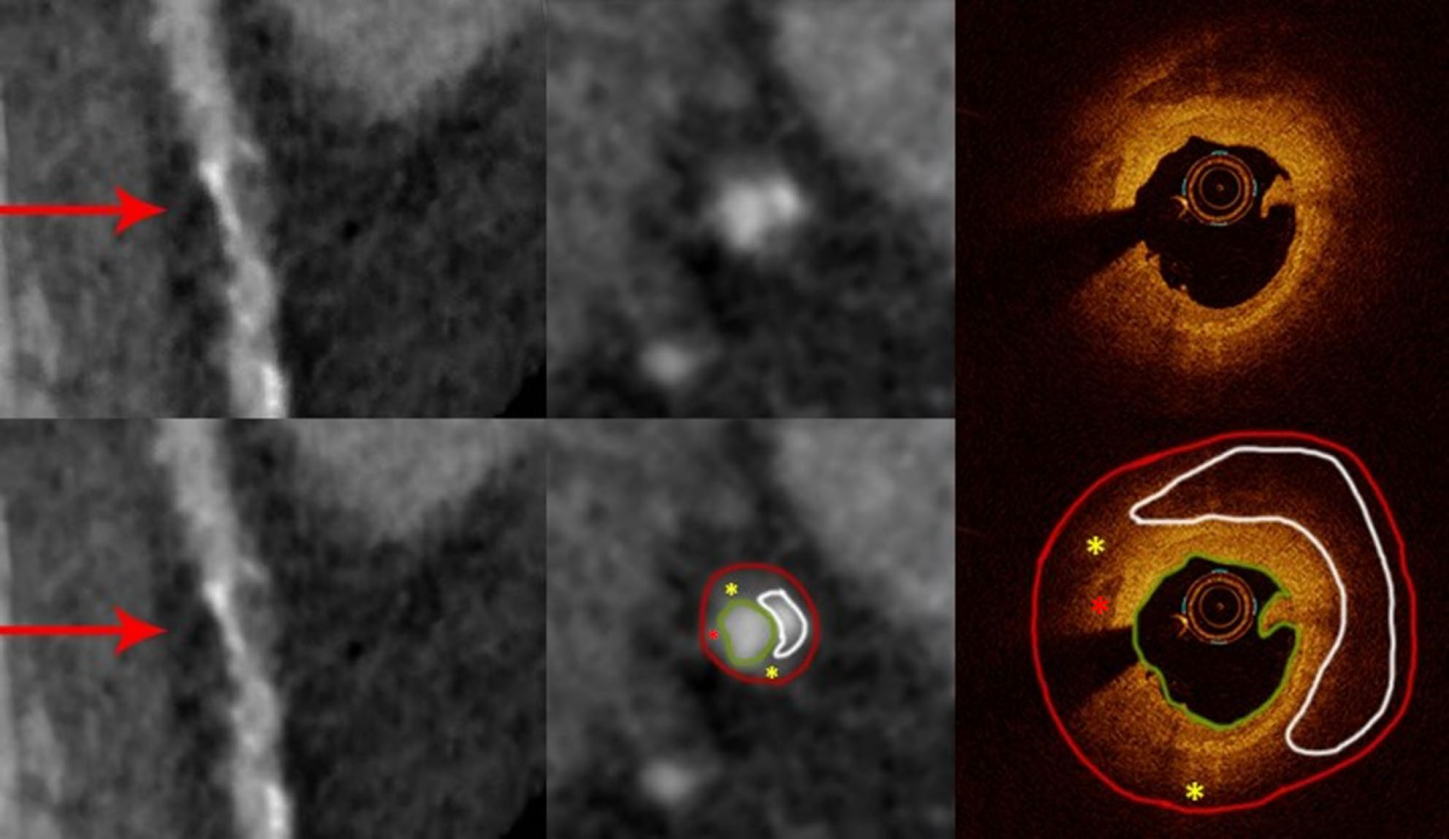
Curved multiplanar reformation computed tomographic images cross-sectional (middle) views of the left anterior descending artery from CCTA (red arrows) demonstrate a mixed plaque with hyperattenuated signal representing the calcified lesion and hypoattenuated signal representing the non-calcified lesion. OCT of the corresponding area shows luminal irregularity and thrombus, with 180 degree of calcium (white outline) with an additional small calcification (red asterisk), and surrounding areas of fibro-lipid plaque (yellow asterisks). The annotations on OCT are applied to the CCTA cross-sectional view (lower middle) where the calcified lesion (white outline) with an additional small calcification (red asterisk), and the surrounding area of non-calcified lesion (yellow asterisks) are matched (lower middle). The red contours encompass the interpolated vessel wall and the green ones outline the vessel lumen.
